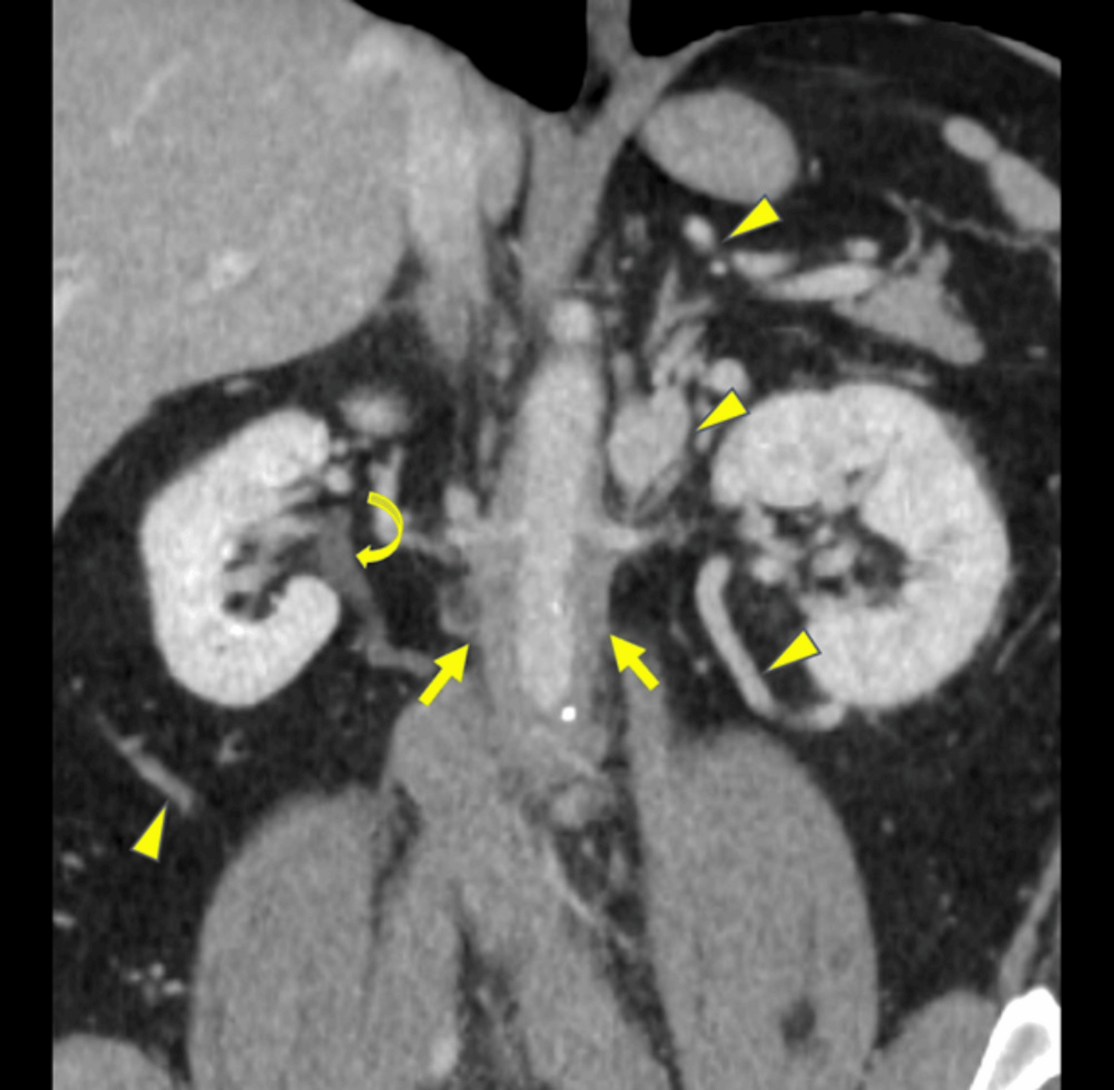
Coronal CT image shows periaortic soft tissue (bold arrows), severe compression of IVC (not seen) with multiple retroperitoneal and subcutaneous venous collaterals (arrowheads), compressing the ureters and right hydronephrosis (curved arrow)
